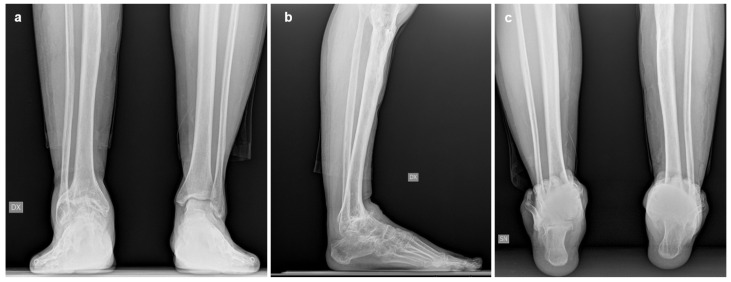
Preoperative weight-bearing radiographs of Case one: (a) anteroposterior view; (b) lateral view; (c) Saltzman view.
